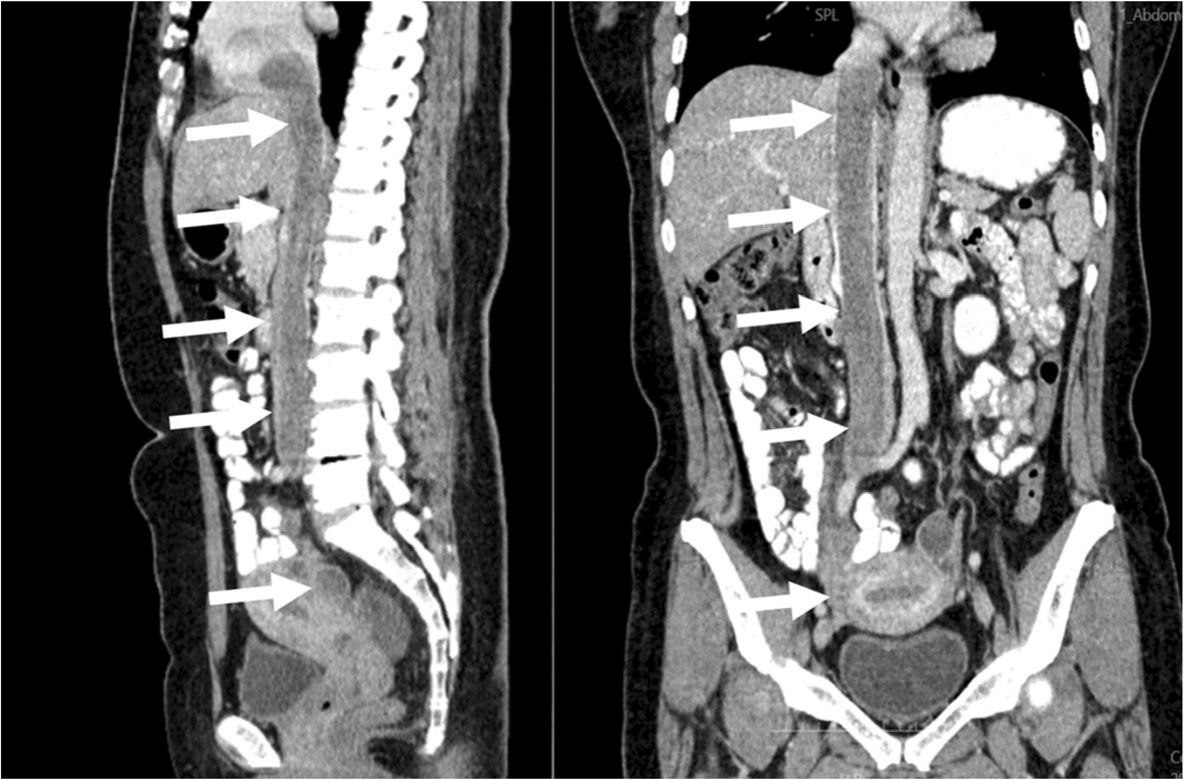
Sagittal and coronal slices of the CT multiplanar reformation show almost the whole appearance and travel path of the tumour; there was a continuous mass coursing through the inferior vena cava to the heart
